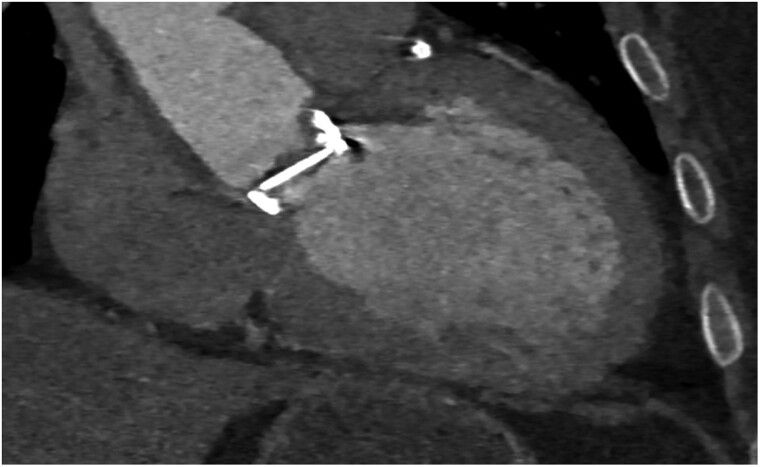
Computed tomography aortogram showing pannus and thrombus in the aortic surface of the metallic aortic prosthesis.
